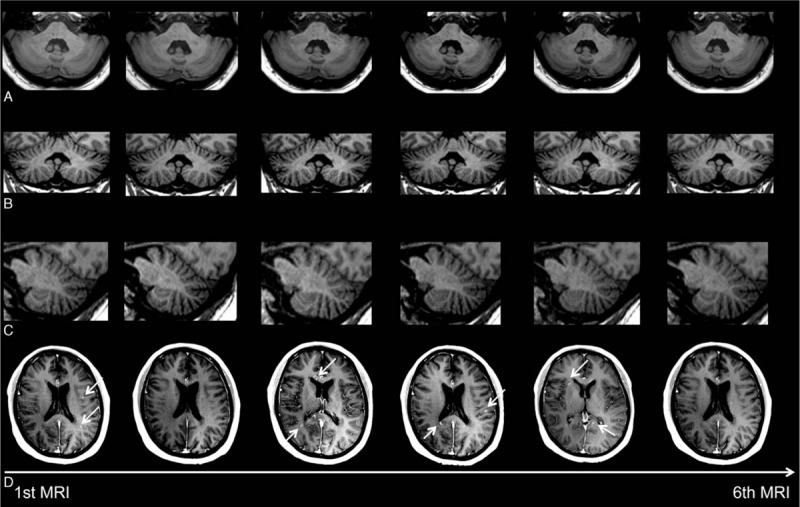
Consecutive (A) axial, (B) coronal, and (C) sagittal unenhanced T1-weighted images of an MS patient at the level of the dentate nucleus. No increase of the signal intensity in the dentate nucleus is observed throughout the examinations. (D) Consecutive MRI examinations of this patient showing multiple contrast-enhancing lesions (white arrows) throughout the 6 examinations.
